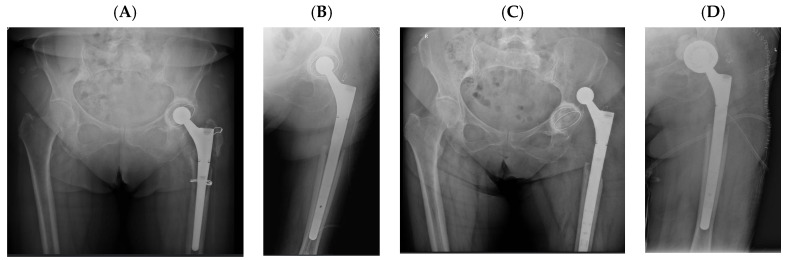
Complex revision case in an adult patient following oncologic resection. (A) Initial radiograph; (B) postoperative image after femoral reconstruction; (C) prosthesis dislocation; (D) revision with DM component.
